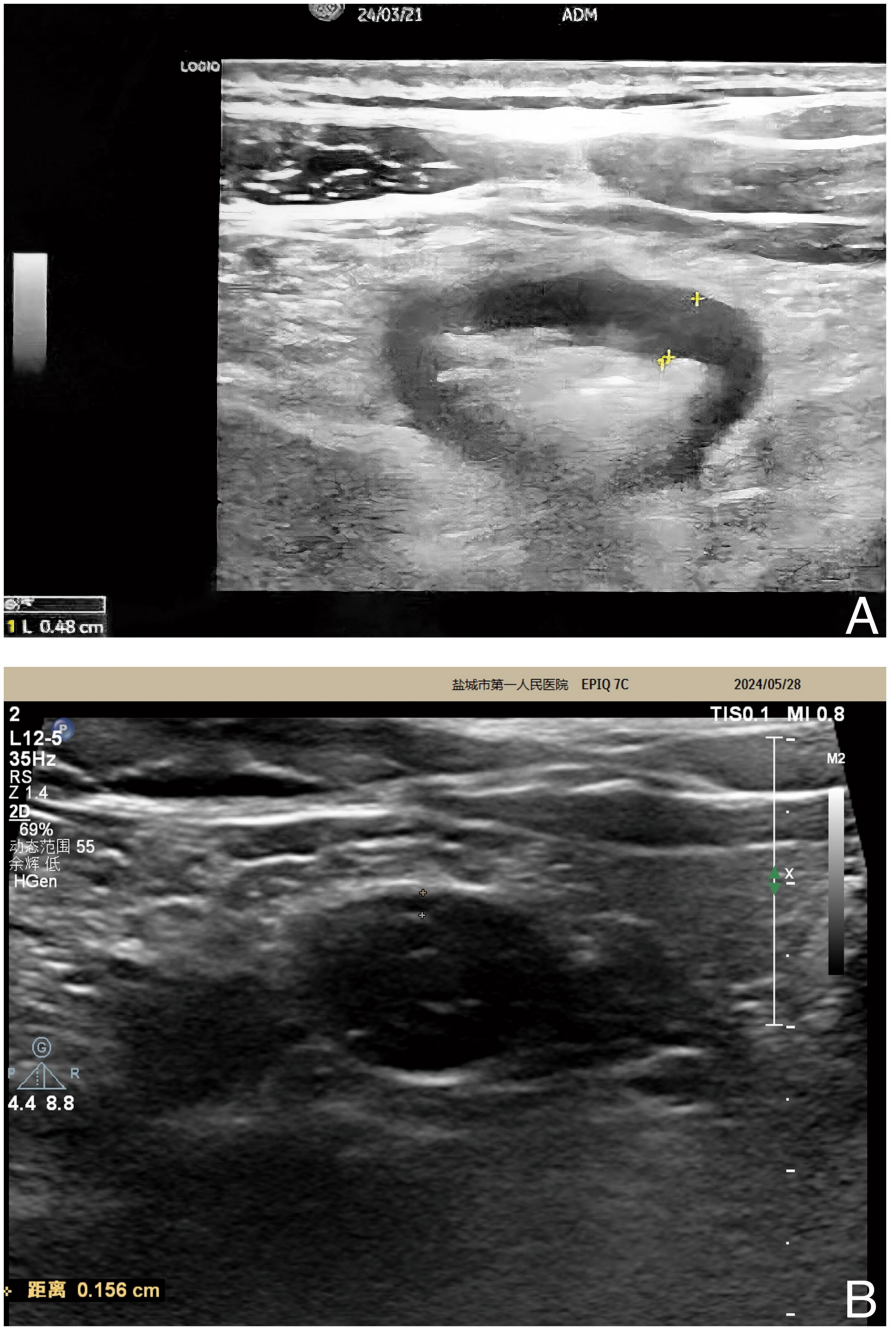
IUS images of the patient. (A) The IUS image prior to the initiation of ACT-U demonstrated a maximum BWT of 4.80 mm. (B) Following a 9-week regimen of ACT-U, the subsequent IUS image reflects a reduction in maximum BWT to 1.56 mm. This notable decrease suggests a positive therapeutic response to the treatment. ACT-U, advanced combination therapy with upadacitinib; BWT, bowel wall thickness; IUS, intestinal ultrasound.
